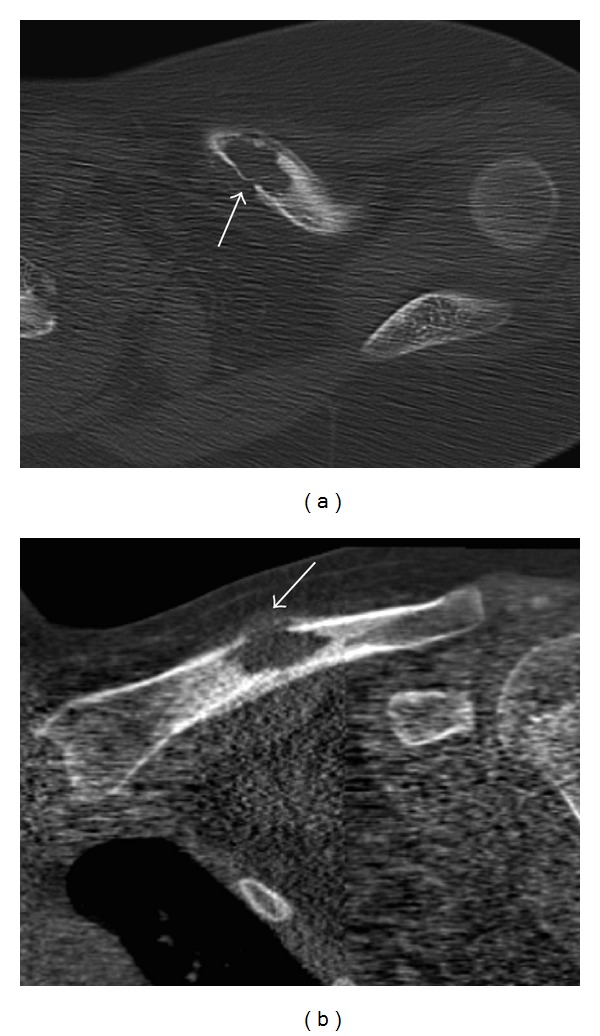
Axial (a) and coronal (b) reformatted CT images in bone window show the lytic mid-clavicle lesion with periosteal reaction and irregular cortical disruption (arrow). No soft tissue mass or marginal sclerosis is detected.
